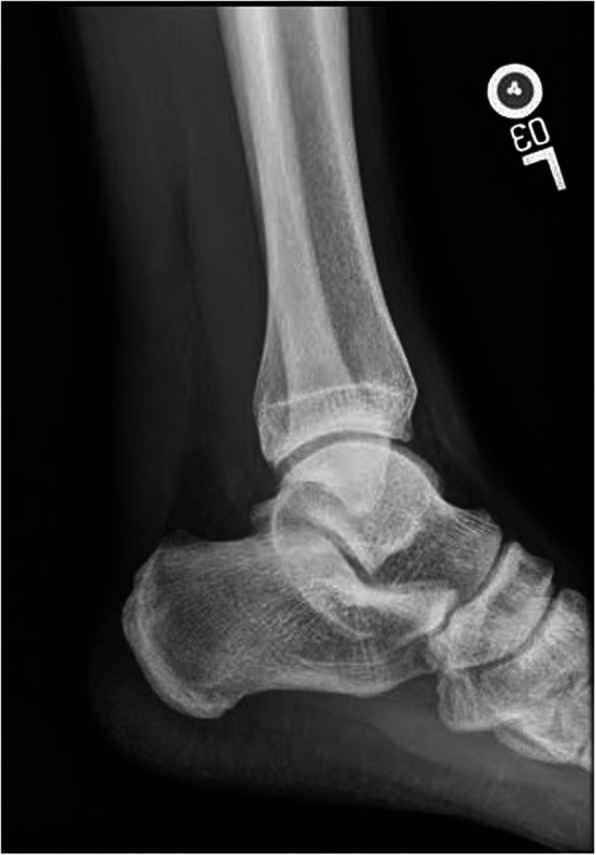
Lateral Radiograph of a left ankle demonstrating disruption of Kager’s triangle
